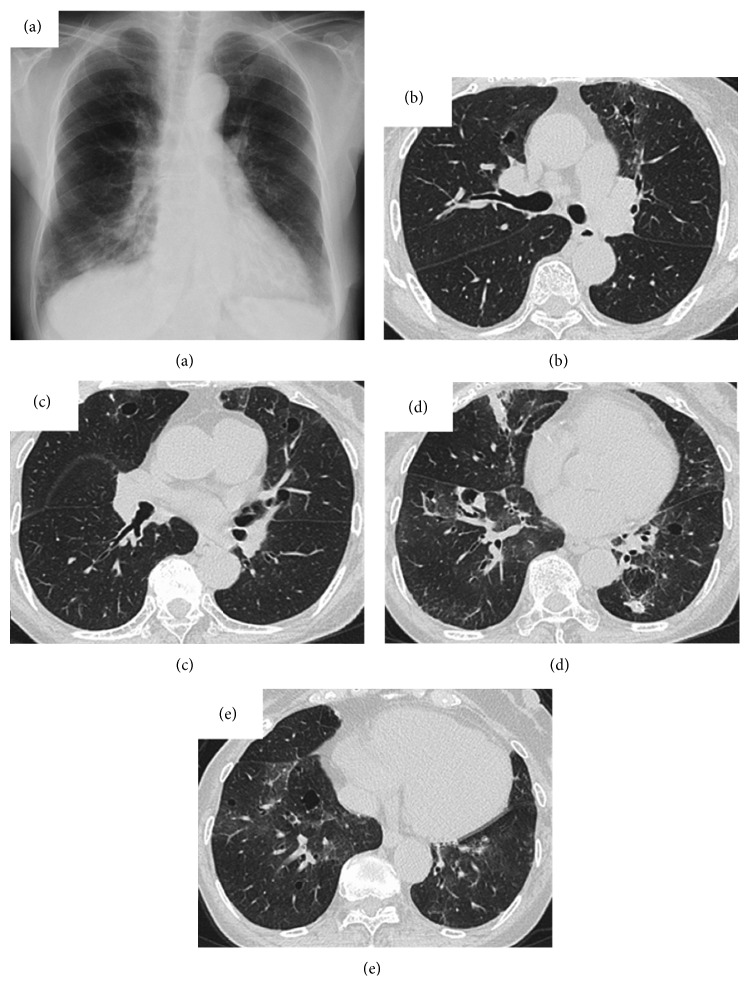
Chest radiograph and CT findings in a 72-year-old woman with Sjögren syndrome and pulmonary amyloidosis. (a) Chest radiograph shows infiltrates in the right lower lung field and ground-glass shadows in the bilateral lower lung fields. Chest CT shows (b, c) multiple cystic lung shadows in the bilateral upper lobes, (d) infiltrates in the right middle lobe and a nodular shadow in the left lower lobe, and (e) ground-glass shadows in the bilateral lower lobes. CT, computed tomography.
